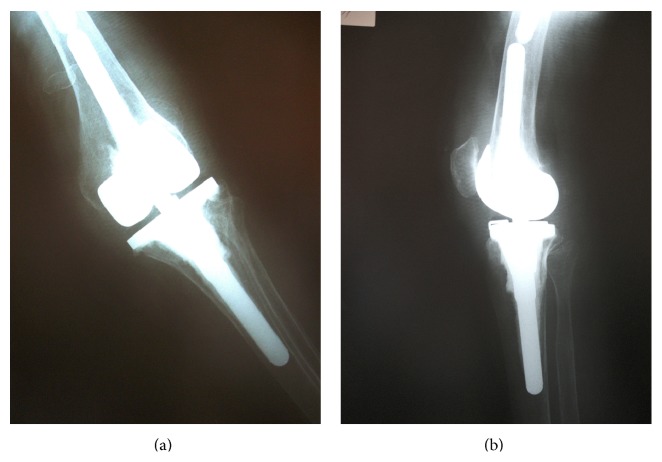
Radiography of the hinged knee prosthesis was satisfactory.
